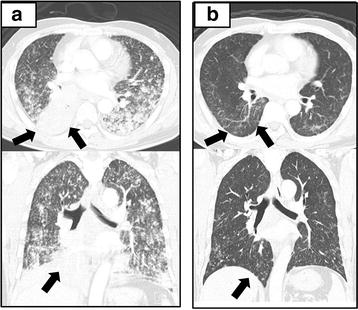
Computed tomography scan of the chest before the initiation of alectinib showing atelectasis in the *right lower* lung and multiple lung metastases (a). Computed tomography scan of the chest 3 weeks after the initiation of alectinib showing improvement of the atelectasis in the *right lower* lung and reduction of multiple lung metastases (b)
